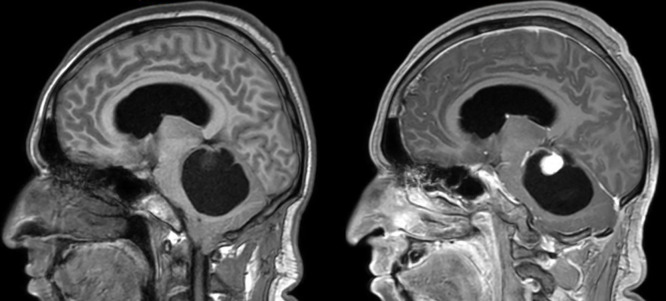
T1-weighted magnetic resonance imaging in the sagittal plane before (left) and after (right) contrast medium administration showing a cystic lesion with an enhancing mural nodule in the cerebellum.
